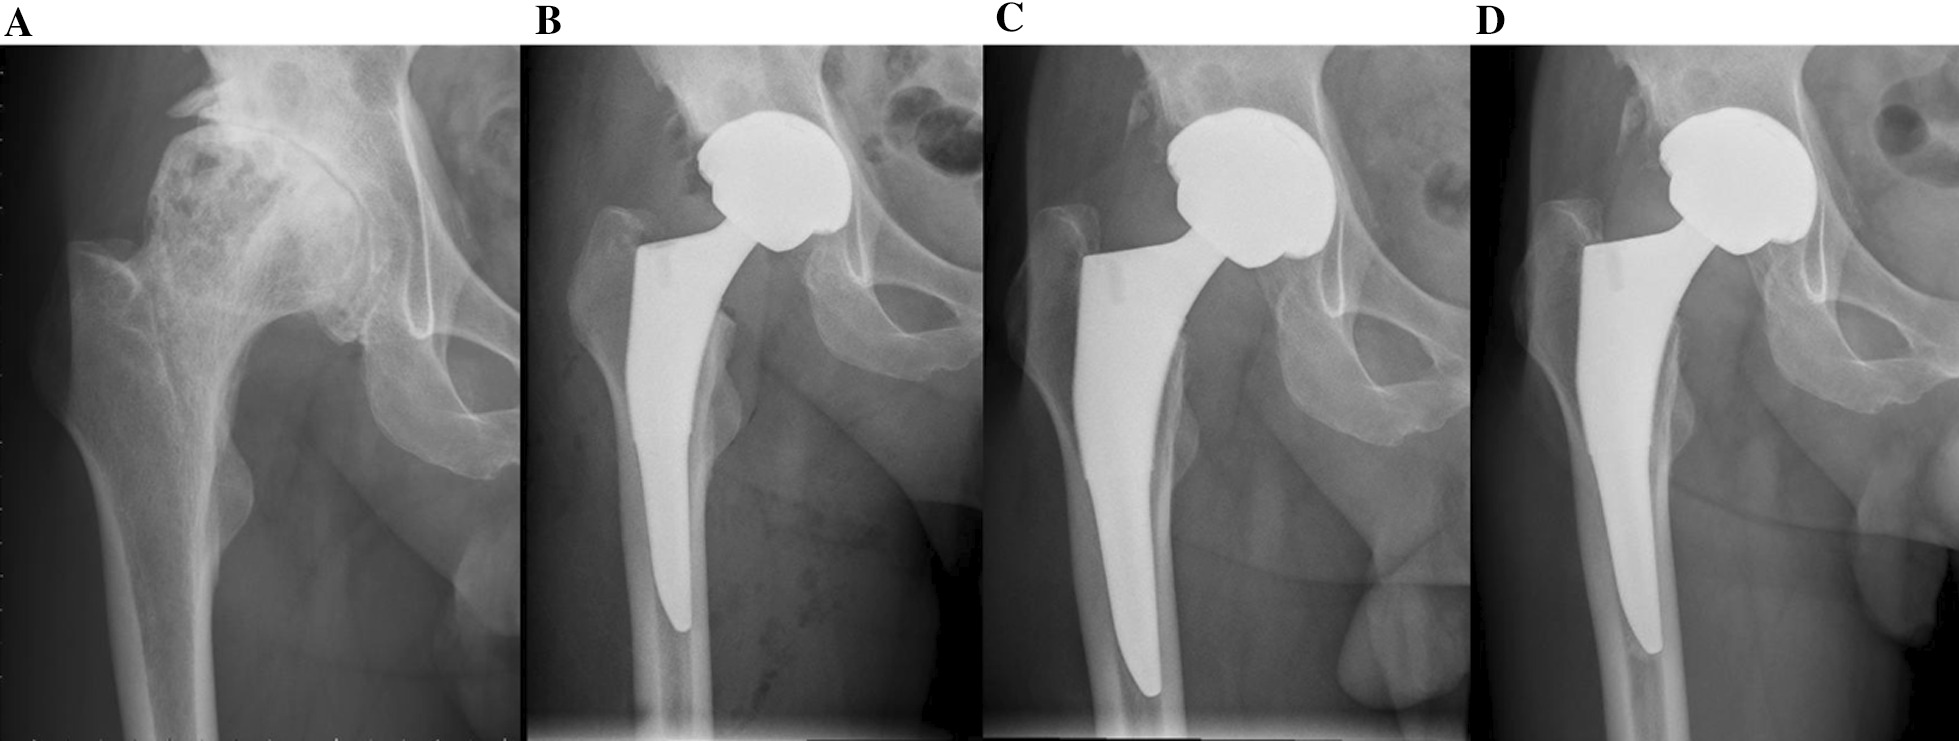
Anteroposterior pelvic radiograph of a 57-year-old male who was diagnosed as OA secondary to infection. The images show the progression of stress shielding at preoperative (a), second day postoperatively (b), 1 year (c), and 4 years post-operatively (d)
